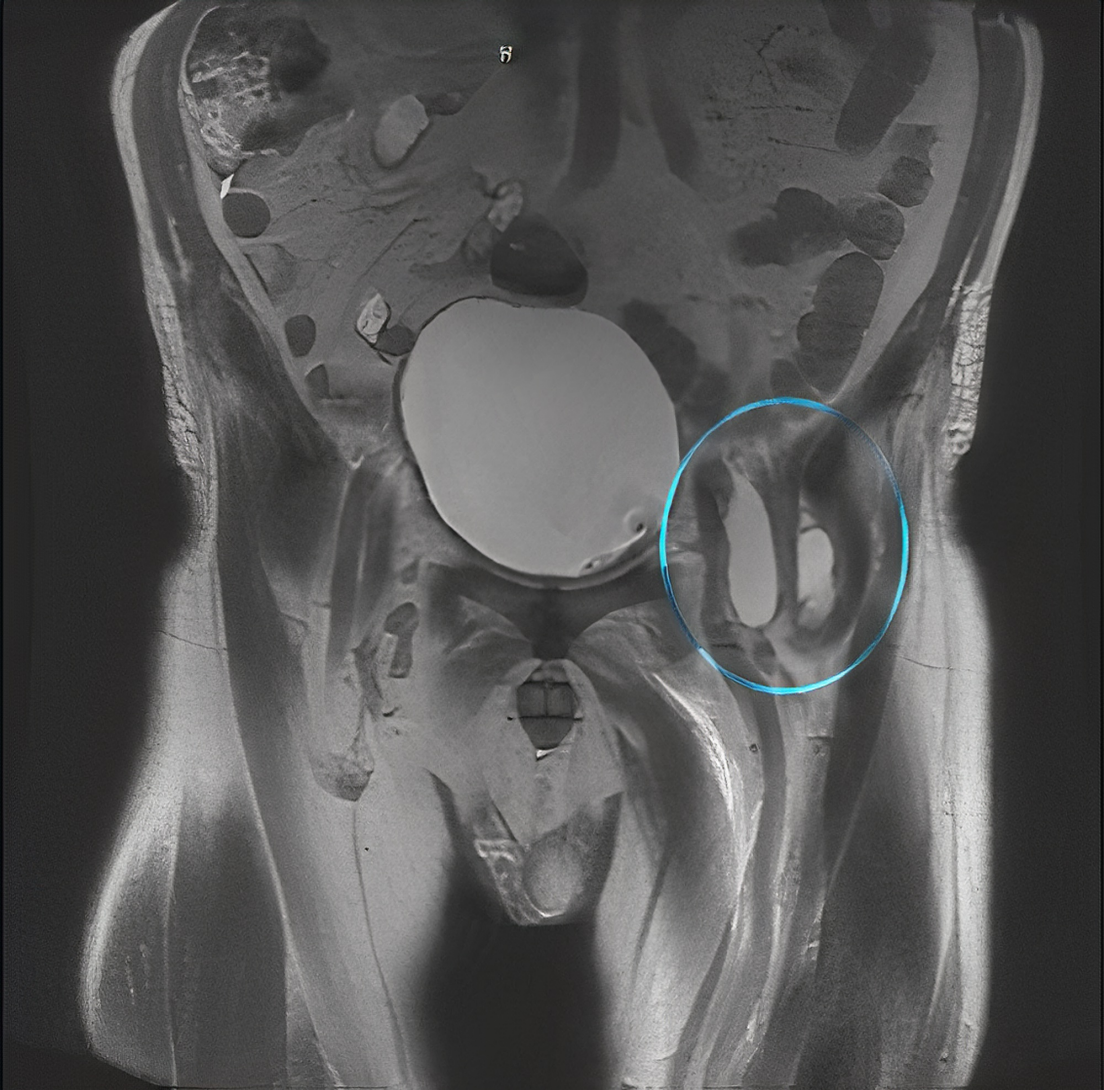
MRI abdomen/pelvis showing abscess extension to the left anterior hip (blue circle).
